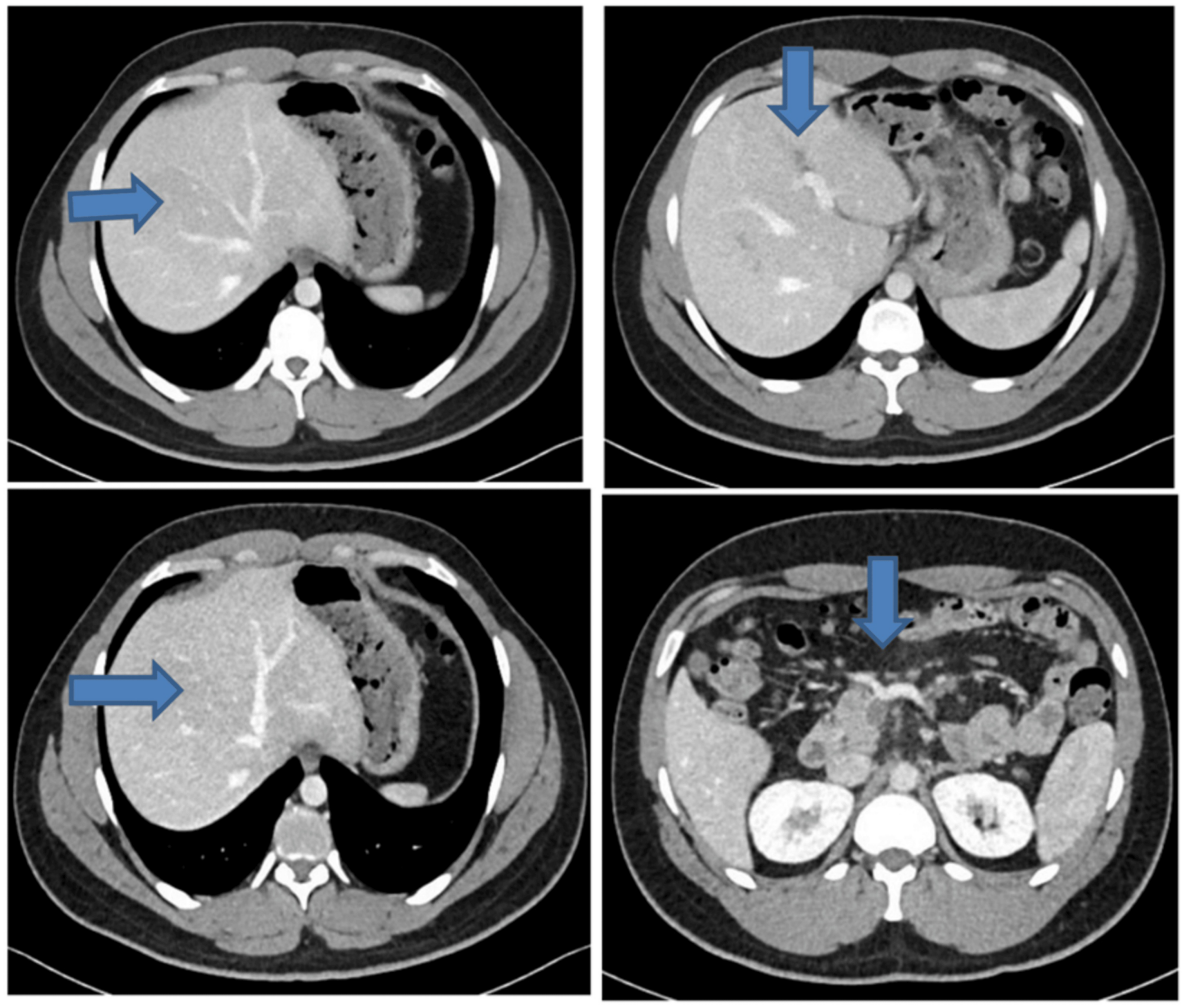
CT scan showing the filling defects of the right branch of the portal vein, extending to the splenic vein and the superior mesenteric vein, with complete non-opacification suggesting acute thrombosis.
